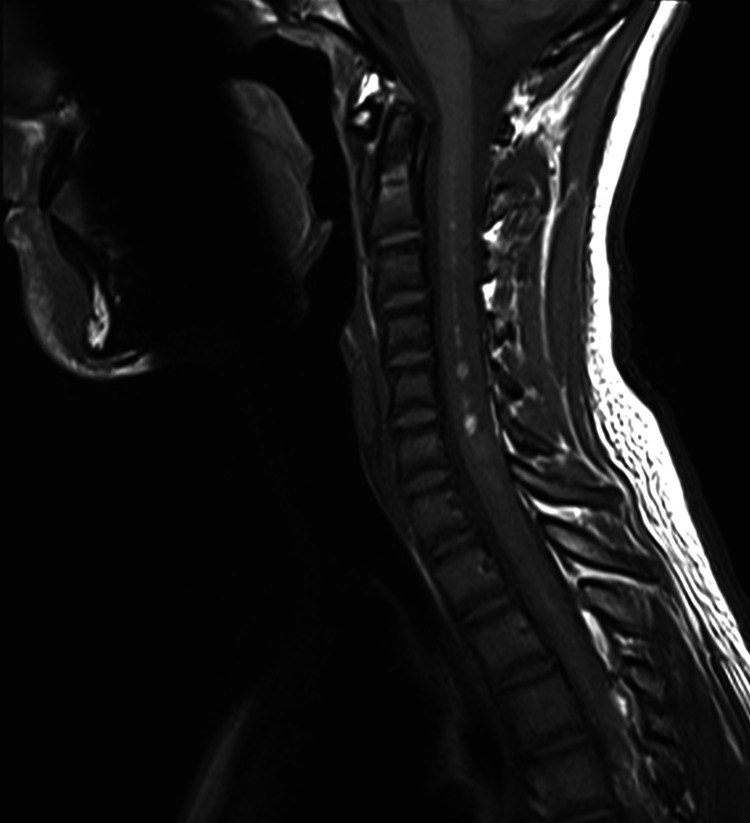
Sagittal T1-weighted MRI of the cervical spine demonstrating a bright intramedullary signal of subacute hemorrhage.
